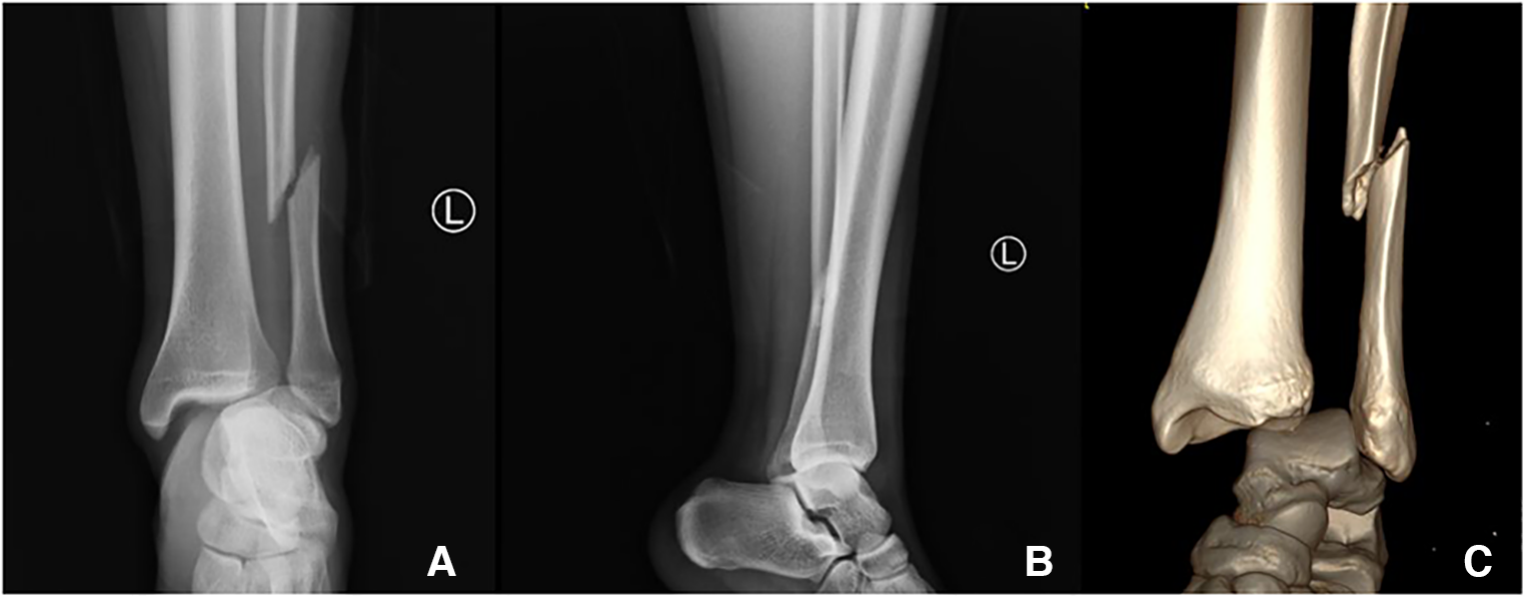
X-ray (A, anteroposterior view and B, lateral view) and 3D reconstruction of a CT scan (C) of a primary left ankle injury. Radiographic evaluation confirmed the diagnosis of a Lauge–Hansen PER stage IV malleolar fracture.
